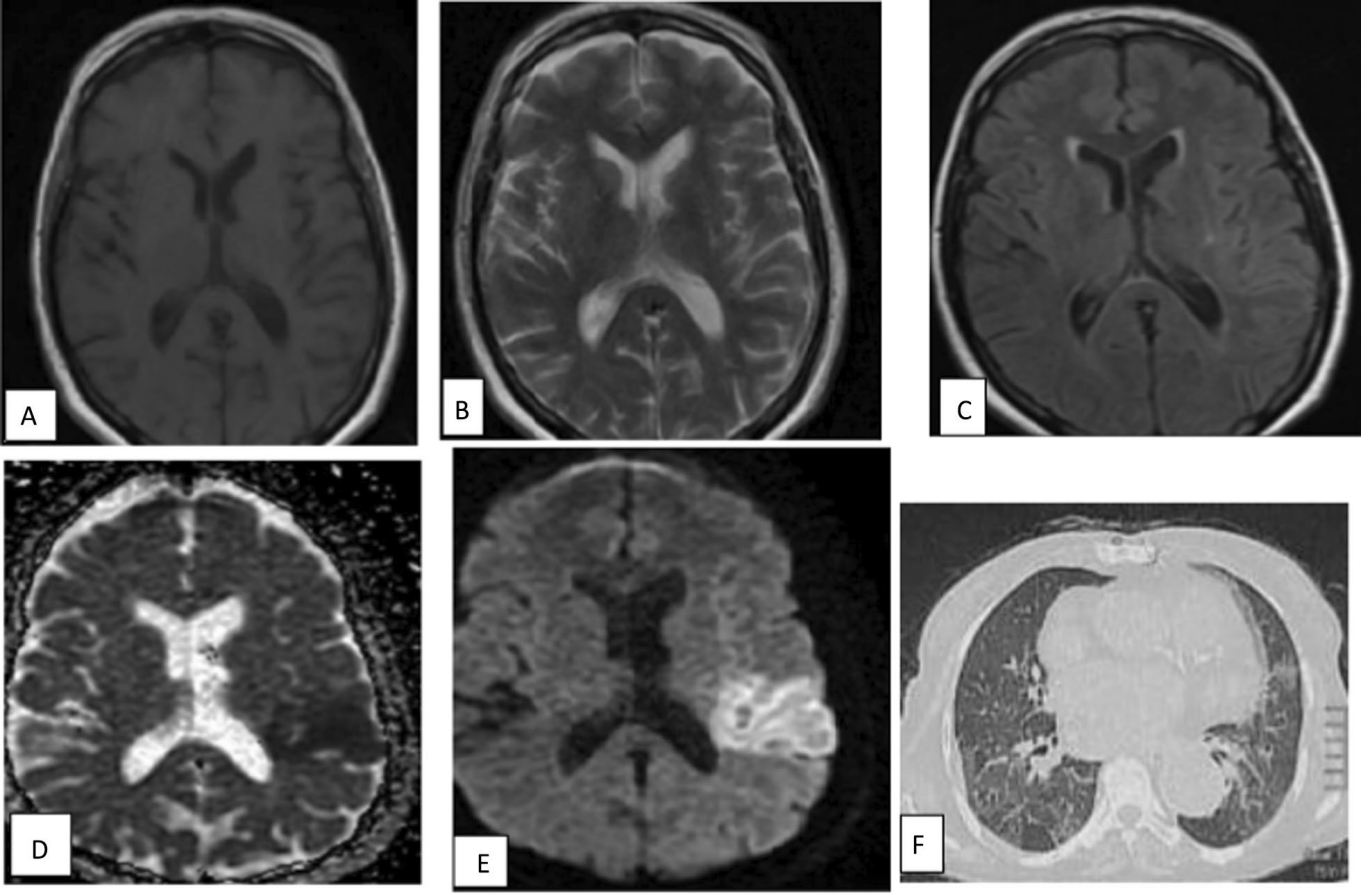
A 50-year-old male patient presented with cerebrovascular stroke post COVID. MRI a axial T1WI, b axial T2WI, c axial FLAIR revealed no abnormality. d ADC map, e DWI revealed a well-defined area of restricted left parietal region acute infarction. f CT chest at admission showing CORAD III (mosaic CT attenuation). CT chest during acute phase is not available
